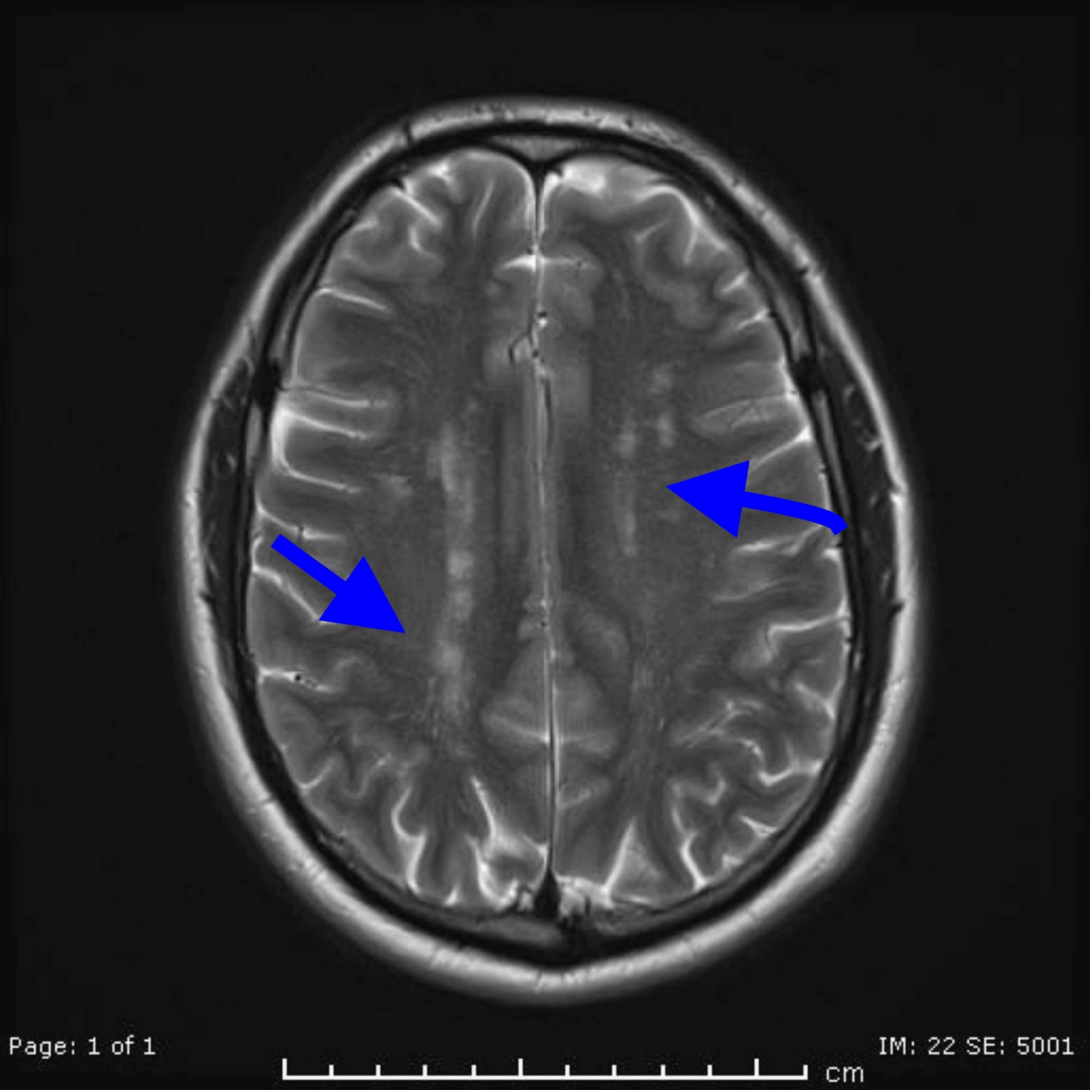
MRI (T2 axial view) of the patient showing findings in keeping with CADASIL CADASIL, cerebral autosomal dominant arteriopathy with subcortical infarcts and leukoencephalopathy
